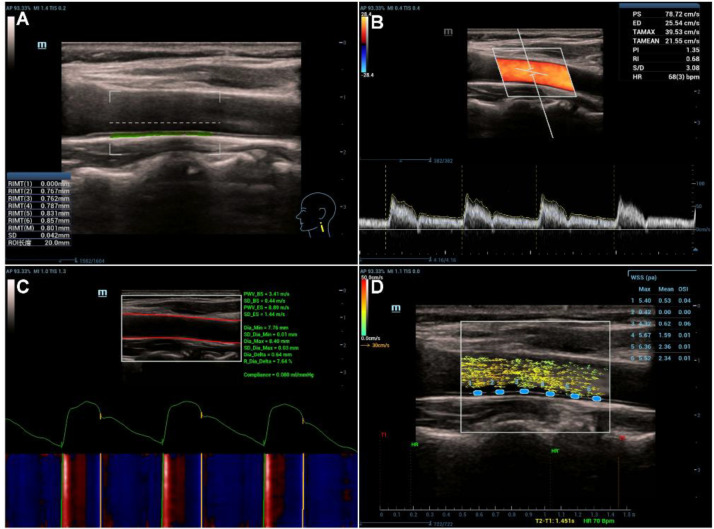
Representative images of carotid vascular assessment using high-frame-rate ultrasound. (A) Automated measurement of the carotid intima-media thickness (cIMT). (B) Hemodynamic assessment of the carotid artery, showing color Doppler flow imaging (top) and the pulsed-wave Doppler spectrum (bottom) for the measurement of peak systolic velocity (PSV) and end-diastolic velocity (EDV). (C) Assessment of arterial stiffness using pulse wave velocity (PWV) technology, showing PWV at the beginning (PWV-BS) and end (PWV-ES) of systole. (D) Vector Flow (V-Flow) mapping for the visualization of complex flow patterns and the quantification of wall shear stress (WSS) within a 20 mm vessel segment.
